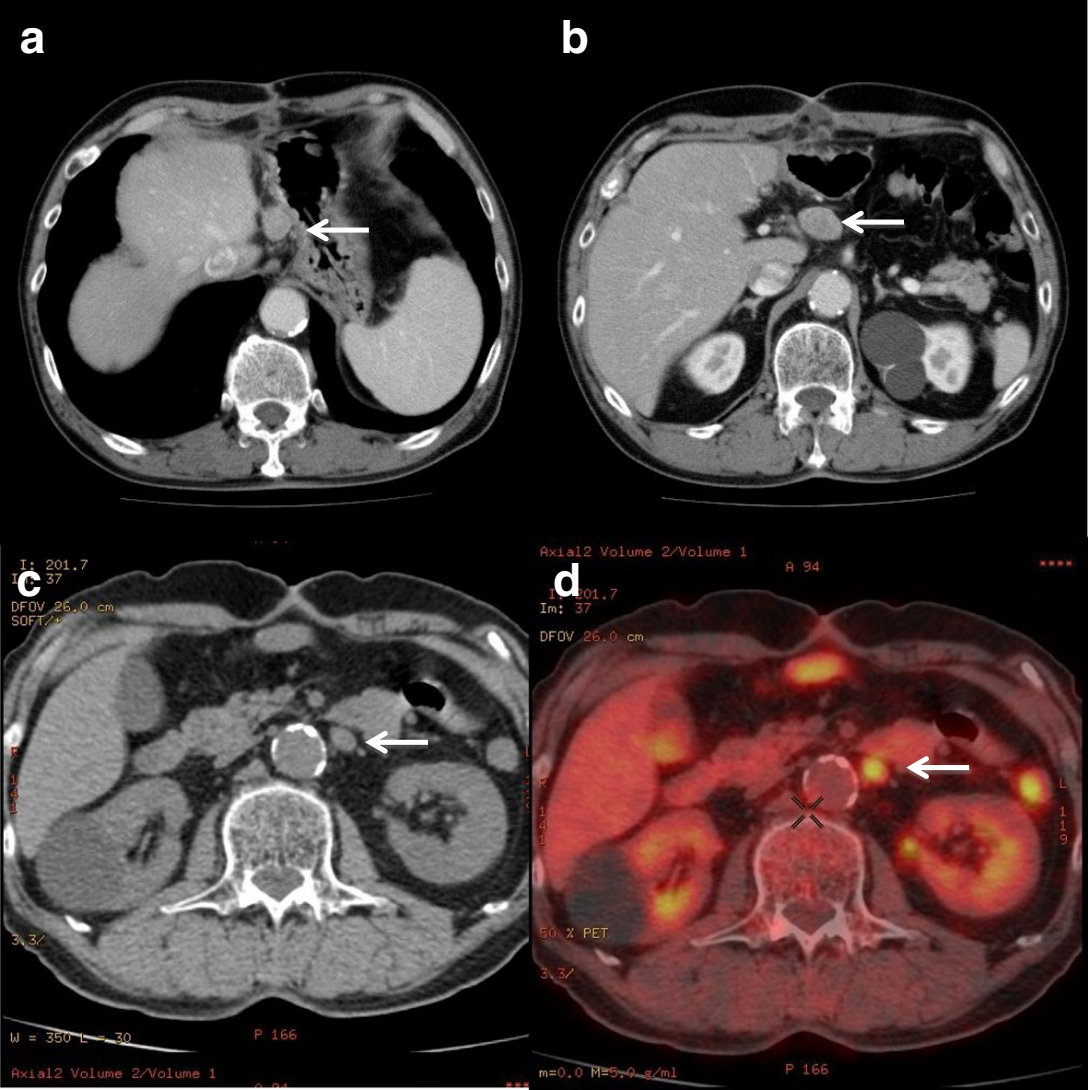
a, b Computed tomography (CT) shows lymph node recurrence (a; #3, b; #8, arrows) . c, d CT and positron emission tomography-CT. c CT shows recurrence (arrow) in the left renal vein lymph nodes. d The SUV max of the tumor was 4.6 (arrow)
